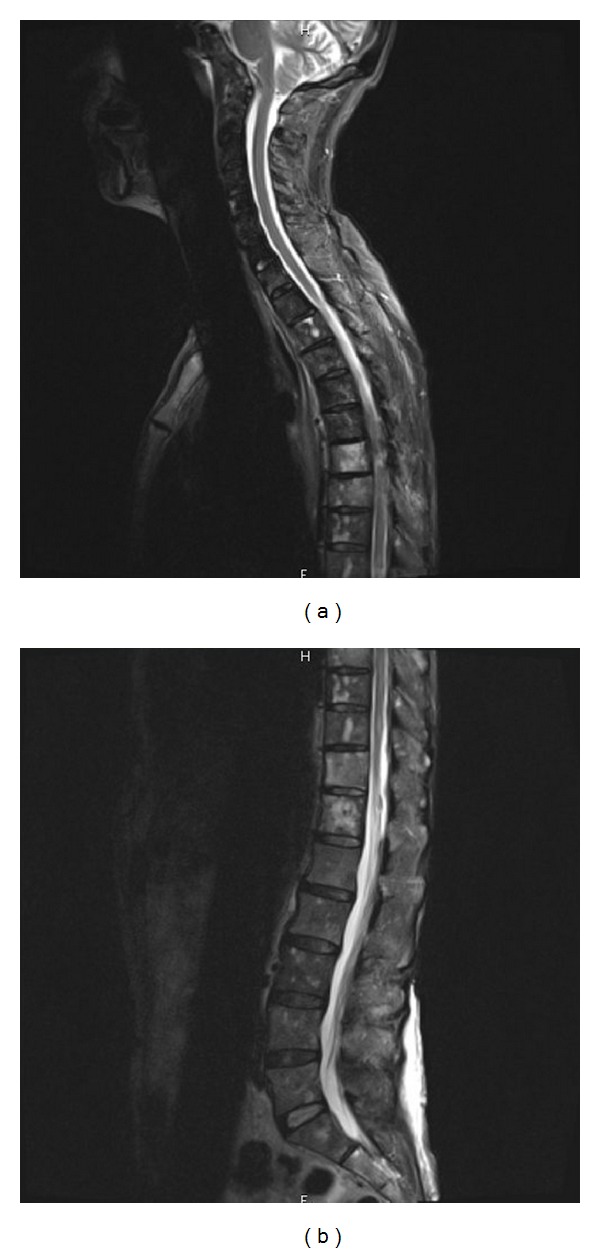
MRI evidence of leptomeningeal carcinomatosis before treatment with IT trastuzumab.
